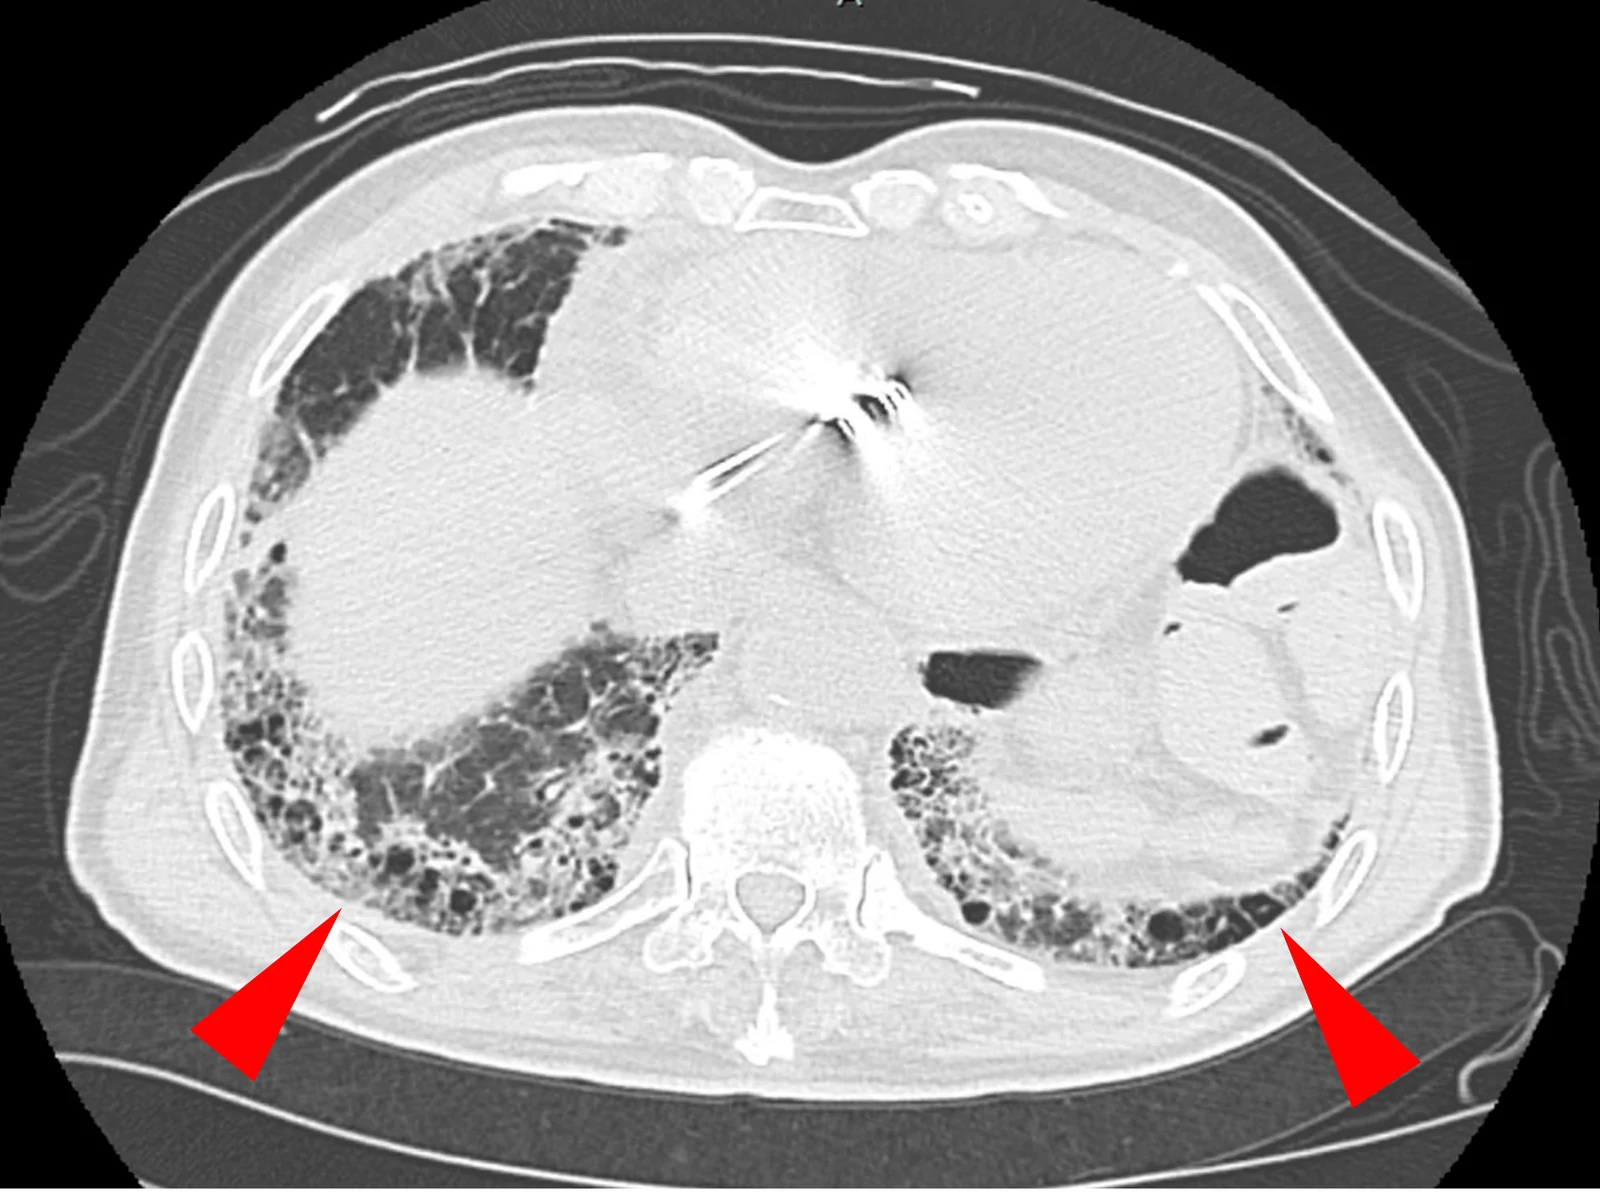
Chest computed tomography on admission Chest computed tomography demonstrating bilateral ground-glass opacities superimposed on pre-existing fibrotic changes, highlighted by red arrowheads
